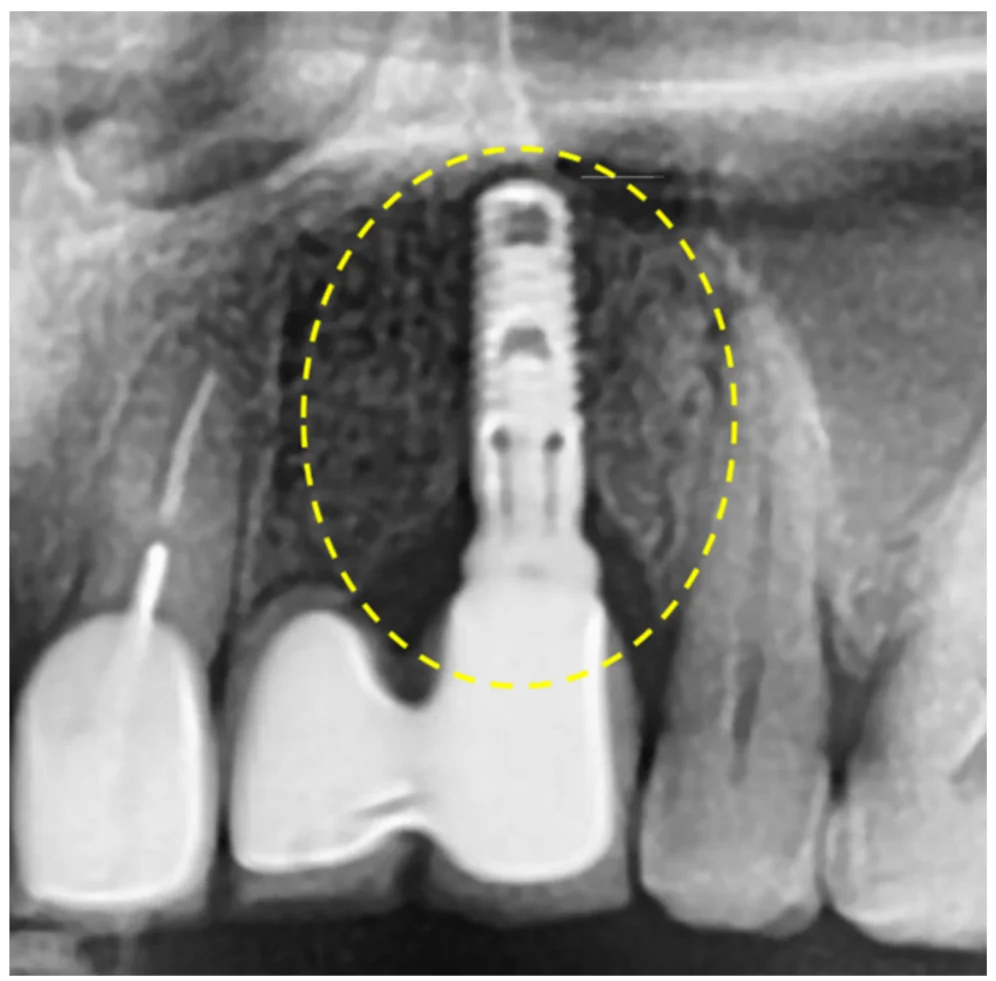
One-year postoperative radiograph demonstrating maintenance of peri-implant bone levels and ridge dimensions.
